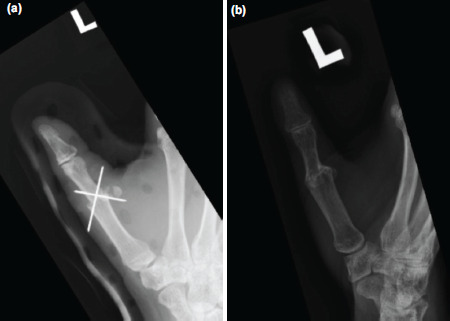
Radiographs of left thumb anteroposterior view (a) day 2 and (b) 1 year post-surgery.
